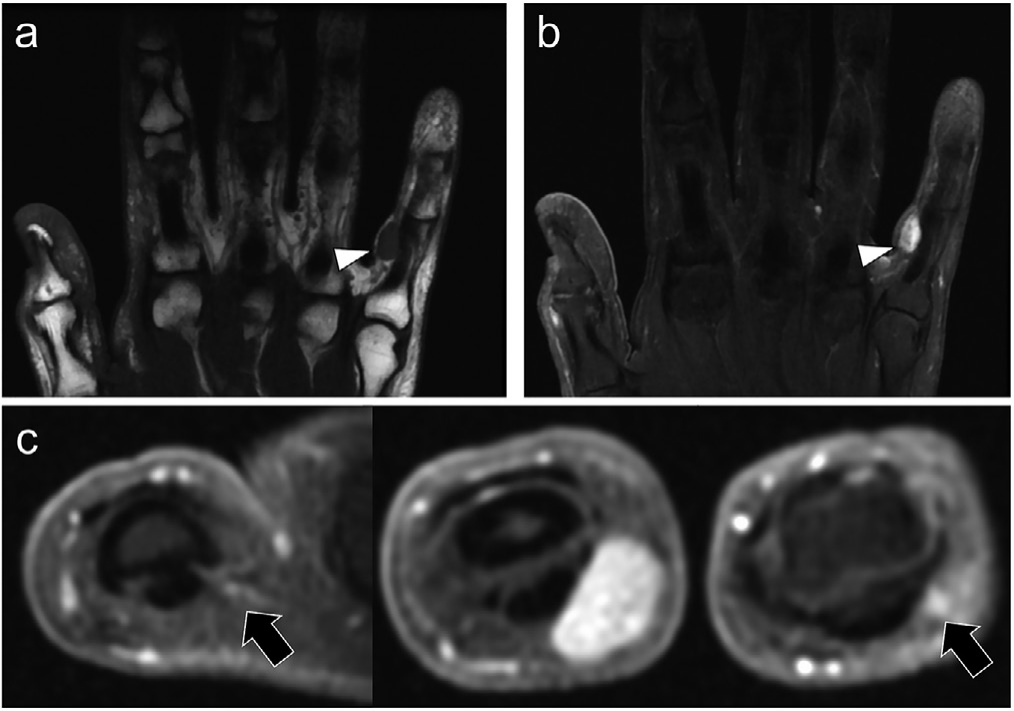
A 51-year-old male underwent a MRI because of a swelling of the fifth finger of the right hand. (a) On coronal *T*_1_WI, the lesion was located on the radial side of the fifth finger. It was 9 mm-long, unique, well-defined, homogeneous and it demonstrated same SIs as normal muscle (white arrowhead). (b) On coronal fat-suppressed *T*_2_WI, the tumor demonstrated homogeneous high SI, without peritumoral edema (white arrowhead). (c) On consecutive axial fat-suppressed, contrast-enhanced *T*_1_WI, the tumor demonstrated marked, homogeneous enhancement, without peritumoral enhancement. It was located in the prolongation of the fifth proper palmar digital vessel (black arrows). SI, signal intensity; *T*_1_WI, *T*_1 _weighted imaging;*T*_2_WI, *T*_2 _weighted imaging.
